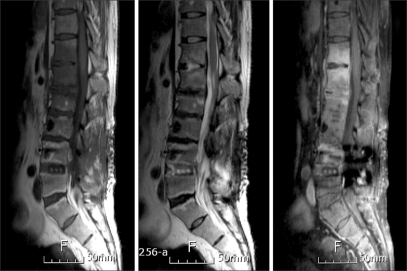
Sagittal T1- and T2-weighted and contrast-enhanced images at 13 months postoperative. MRI showed subligamentous extension of inflammation to the T12-L1 intervertebral disc.
